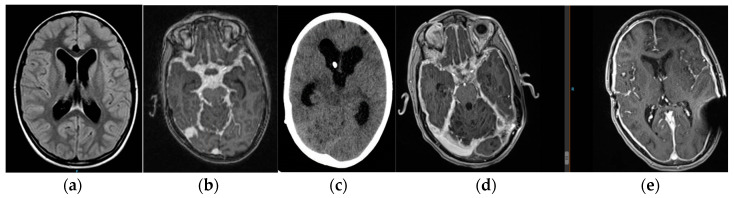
Image (a) shows condition prior to the placement of the first shunt system. Images (b,c) correspond to the moment when the patient was diagnosed with a leptomeningeal oncologic process and developed hydrocephalus, despite pressure reductions. Note the subarachnoid space occupied by tumor content. Images (d,e) show response to systemic treatment with visualization of the cisterns. The last CT even shows meningeal enhancement and laminar ventricles suggestive of hyper drainage.
